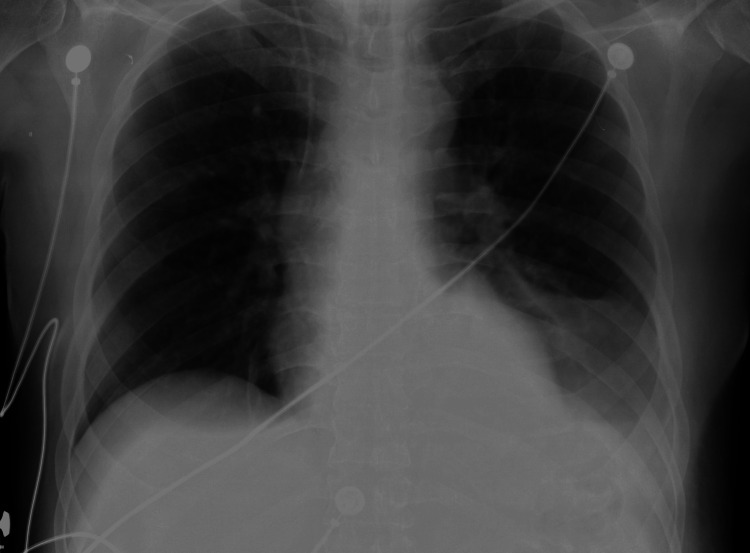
Pre-central venous catheterization (CVC) insertion chest X-ray
